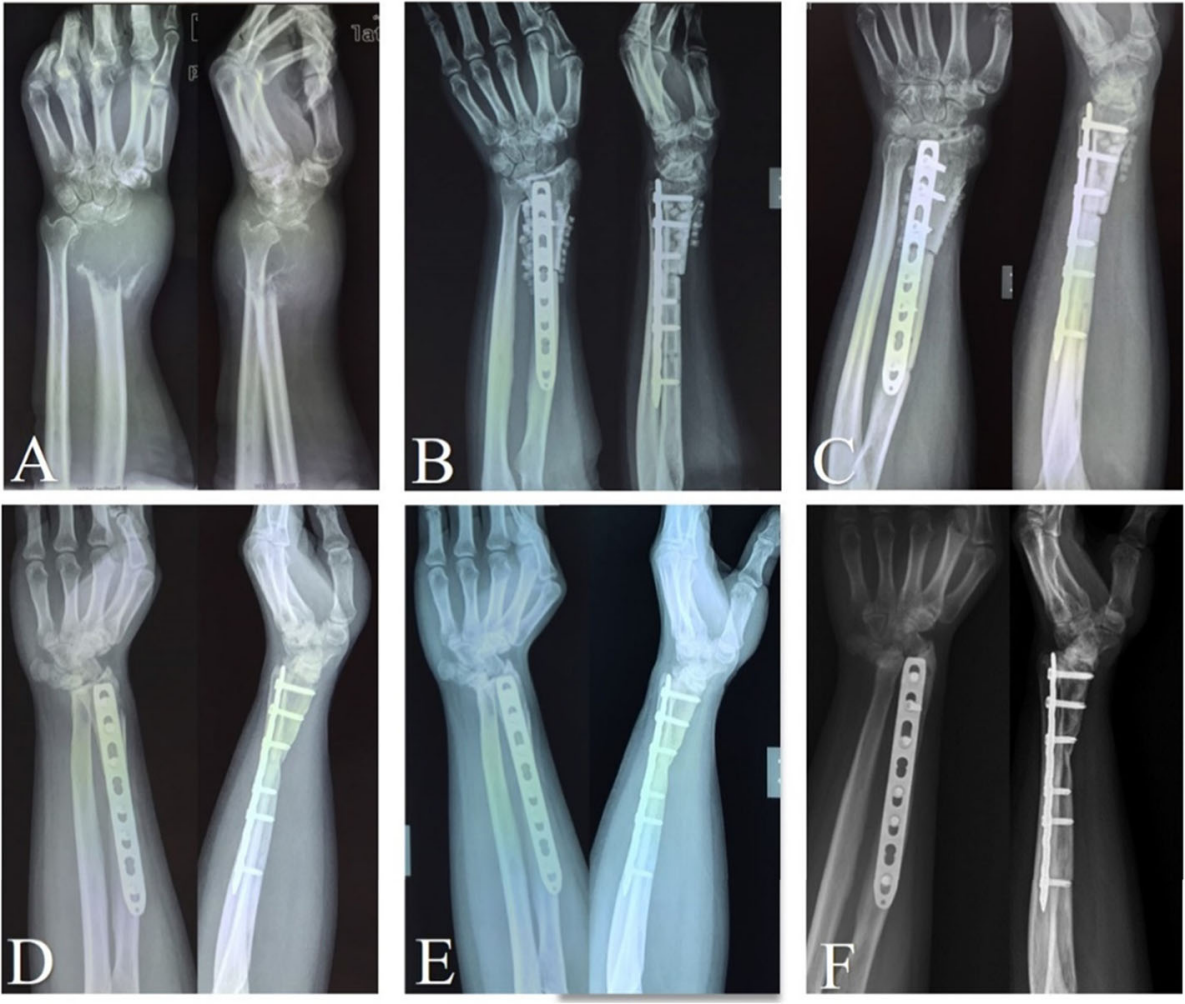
The serial X-rays show the nature of radiological degeneration with allograft reconstruction. A: before surgery; B: 2 days after surgery; C: 2 months after surgery; D: 10 months after surgery; E: 24 months after surgery; F: 54 months after surgery
